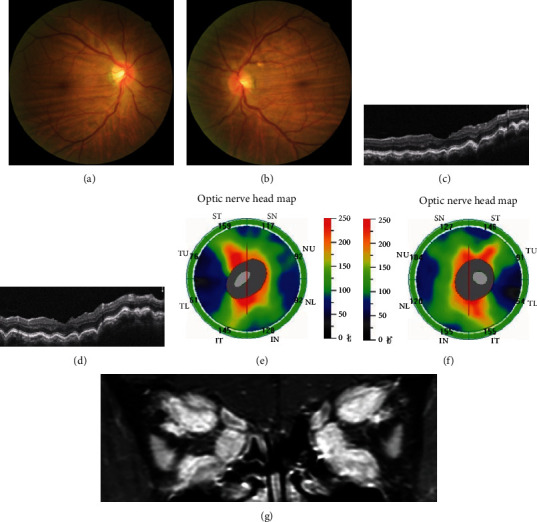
TAO patient of a 57-year-old male who presented with bilateral DON and CRFs. (a, b) The fundus photograph of bilateral CRFs. (c, d) The OCTA of bilateral CRFs in macula. (e, f) The OCTA of bilateral CRFs in optic nerve head; (g) MRI represents the crowded orbital apex and phlegmonosis from the enlarged extraocular muscles.
